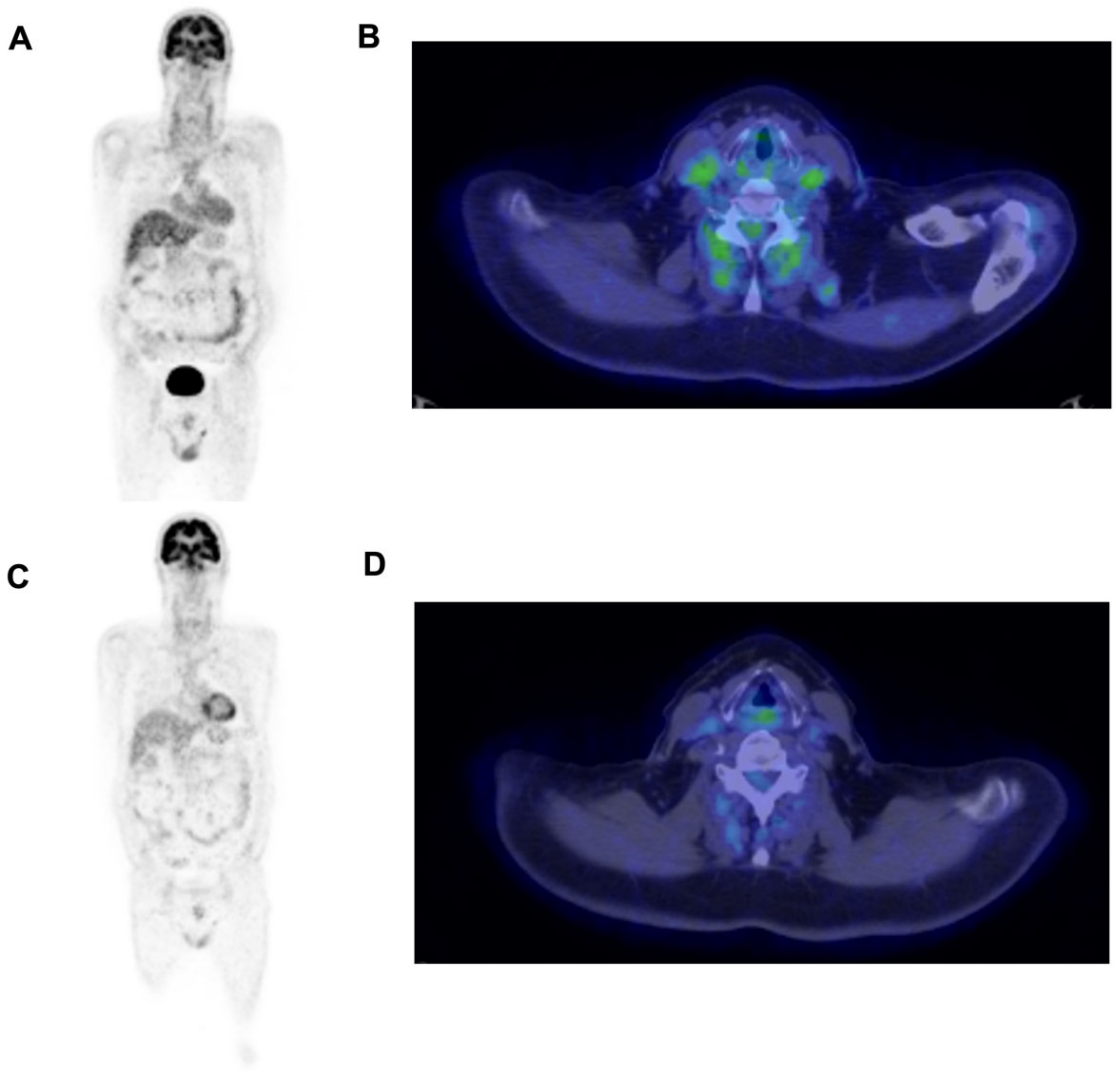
^18^F-FDG PET/CT scan at disease onset and after 3 years (A, B)
